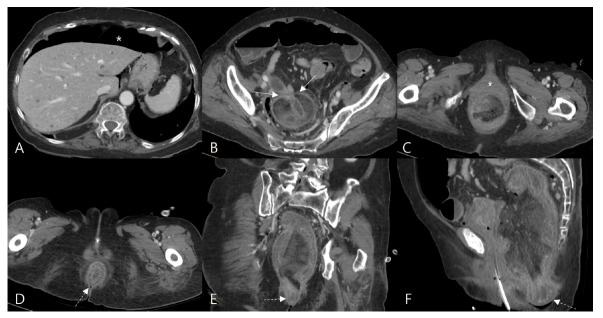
Pre- and post-contrast-enhanced abdominopelvic CT images in an 81-year-old woman. Axial contrast-enhanced CT images (A-D), coronal reformatted image (E) and sagittal reformatted image (F). Axial image (A) shows a large amount of intraperitoneal free air (white asterisk). Axial image (B) demonstrates a large wall defect (white arrows) in the rectum, with small bowel loops and mesentery herniating into the rectal lumen. Axial image (C) shows herniated small bowel and mesentery in the anal canal. Axial, coronal and sagittal images (D-F) reveal small bowel loops (white dashed arrows) protruding externally beyond the anal verge, suggestive of transanal evisceration. The herniated bowel loops exhibit diffuse edematous wall thickening with decreased bowel wall enhancement, highly suspicious of strangulation.
